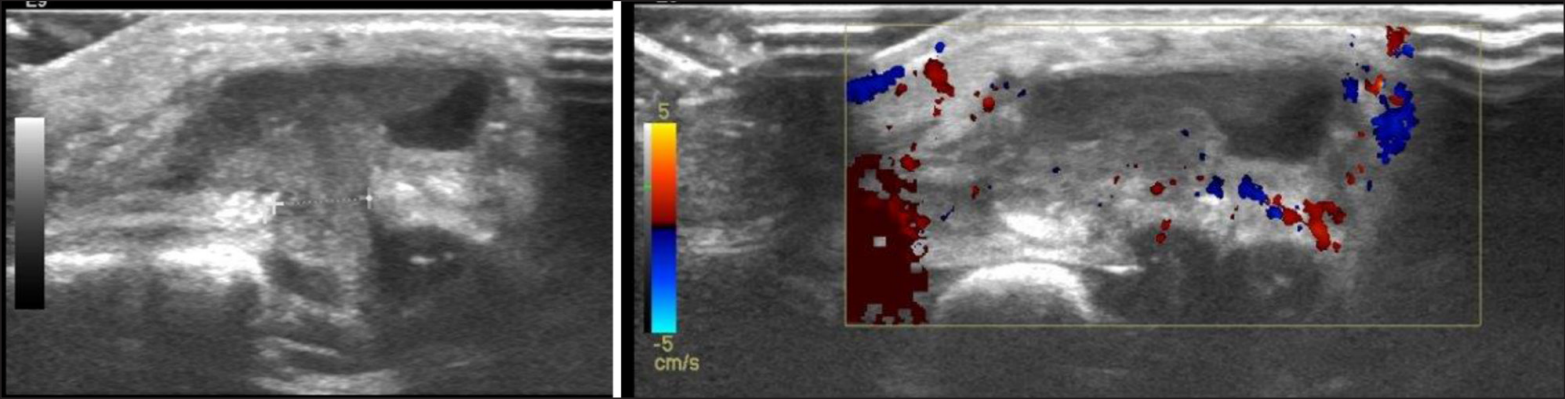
Ultrasonography shows a sharp defined lesion with a neck and presternal component (dumbbell sign) and is mainly hypo-echoic in comparison to the subcutaneous fat tissue. There is no internal vascularisation.
